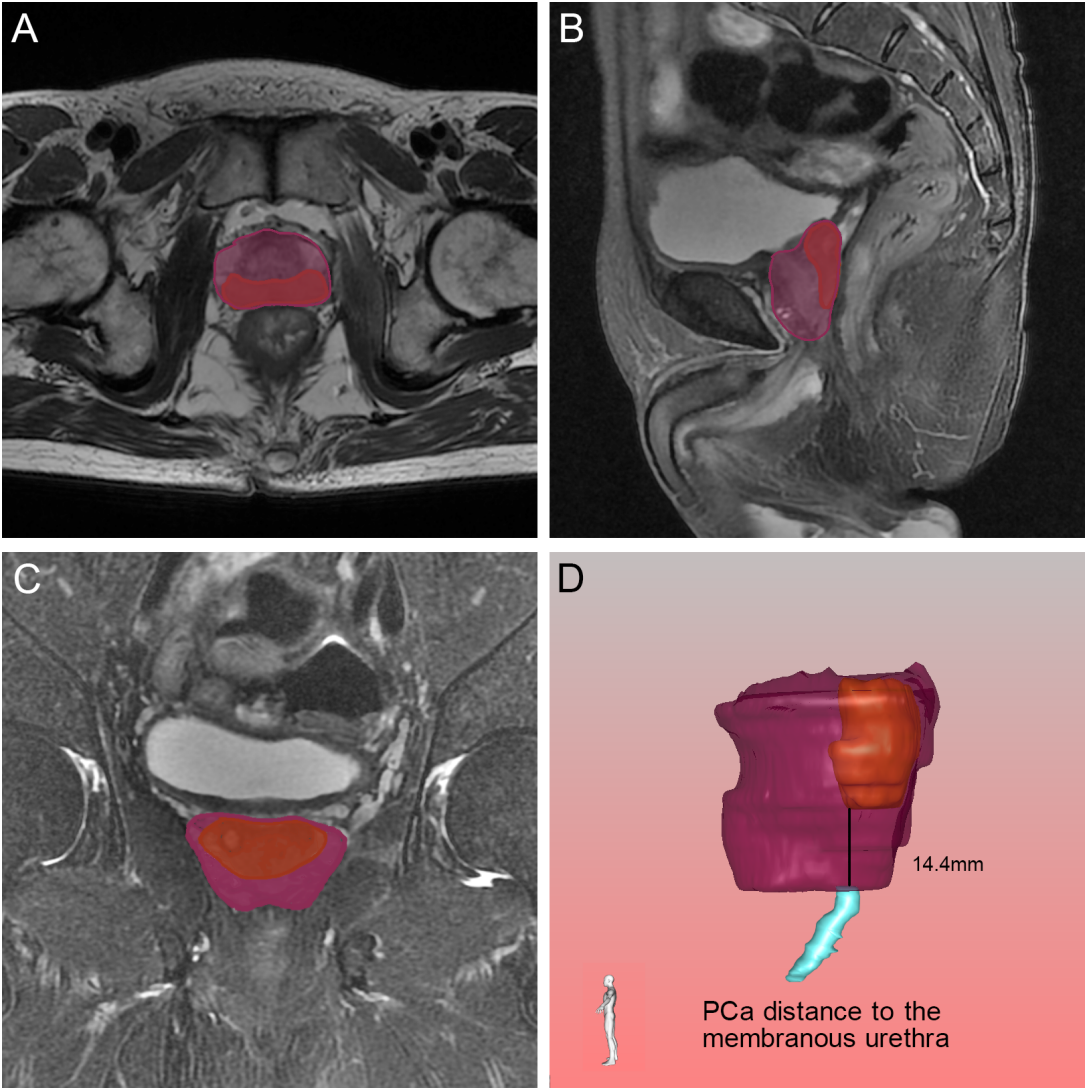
Relative position of the tumor to the membranous urethra in axial (A), sagittal (B) and coronal (C) views on magnetic resonance imaging, and the black line demonstrated the PCa distance to the membranous urethra found by the three-dimensional visualization technique (D). The orange, blue and purple sections indicated the prostate cancer, membranous urethra and prostate respectively.
